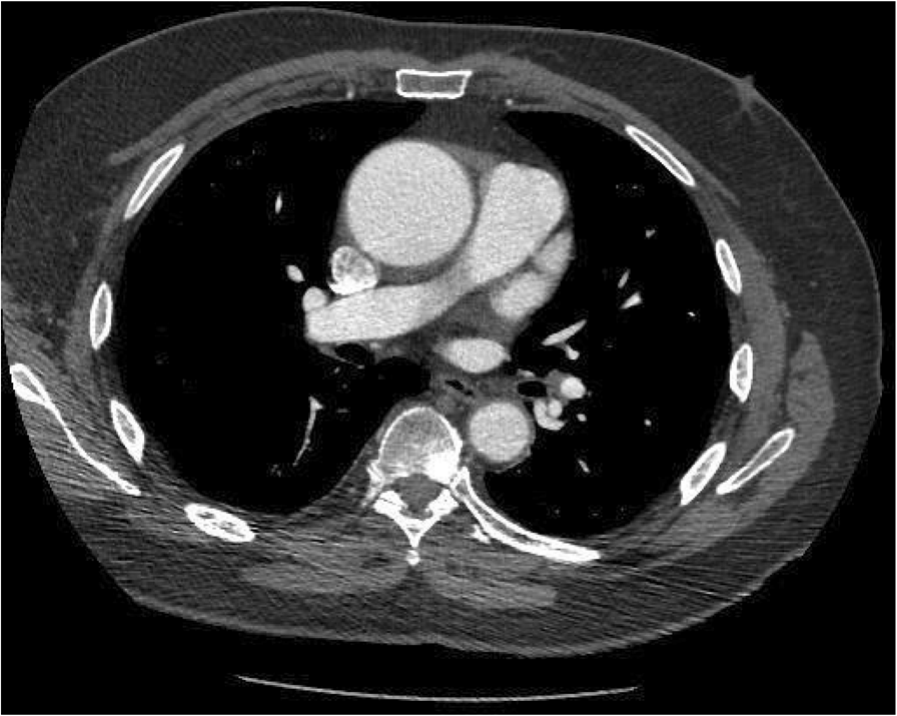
CT Aorta showing aortic dilatation. No signs of dissection
